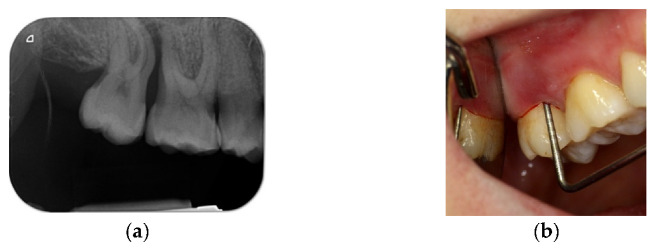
(a) Periapical radiograph of teeth 1.7, 1.6, 1.5; (b) important probing periodontal mesial root of 1.7.
